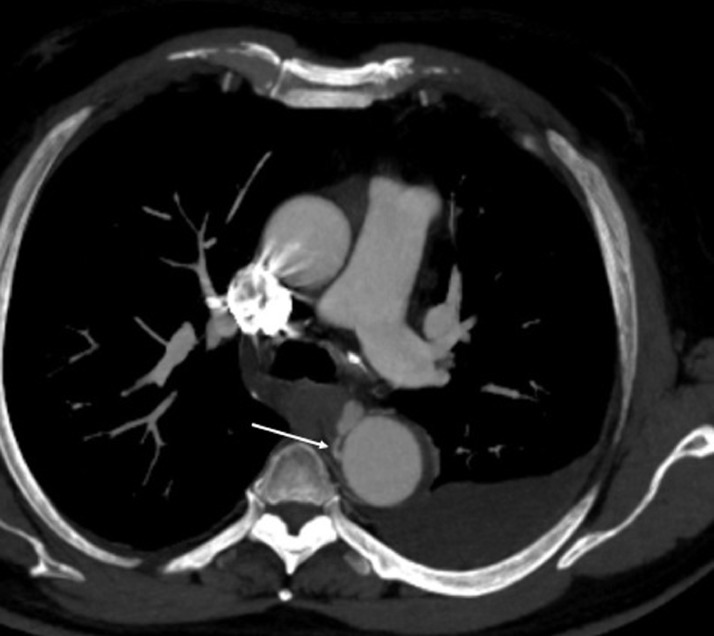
Axial CT scan view in the arterial phase showing the left bronchial artery feeding the aneurysm (arrow).
